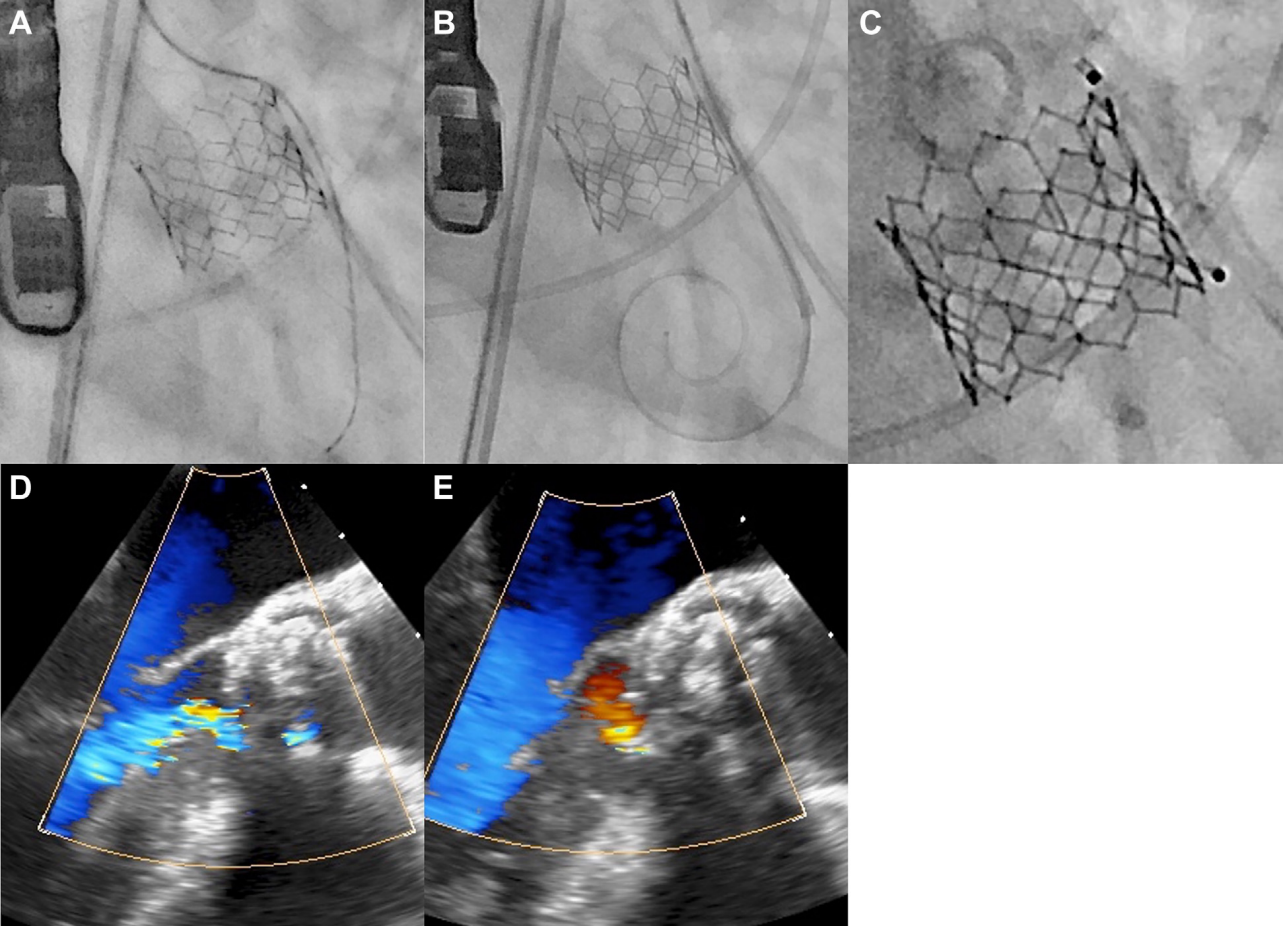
Procedure of Percutaneous Plug Closure (A) A 0.035-inch hydrophilic guidewire with 5-F guiding sheath and 4-F Amplatz-Left-2 catheter passed the gap. (B) Guidewire was exchanged to Safari XS and 6-F straight catheter passed the gap. (C) An 8 × 7-mm Amplatzer Vascular Plug II was implanted. (D) Transesophageal echocardiography of pre-procedure. (E) Transesophageal echocardiography after implantation of vascular plug.
